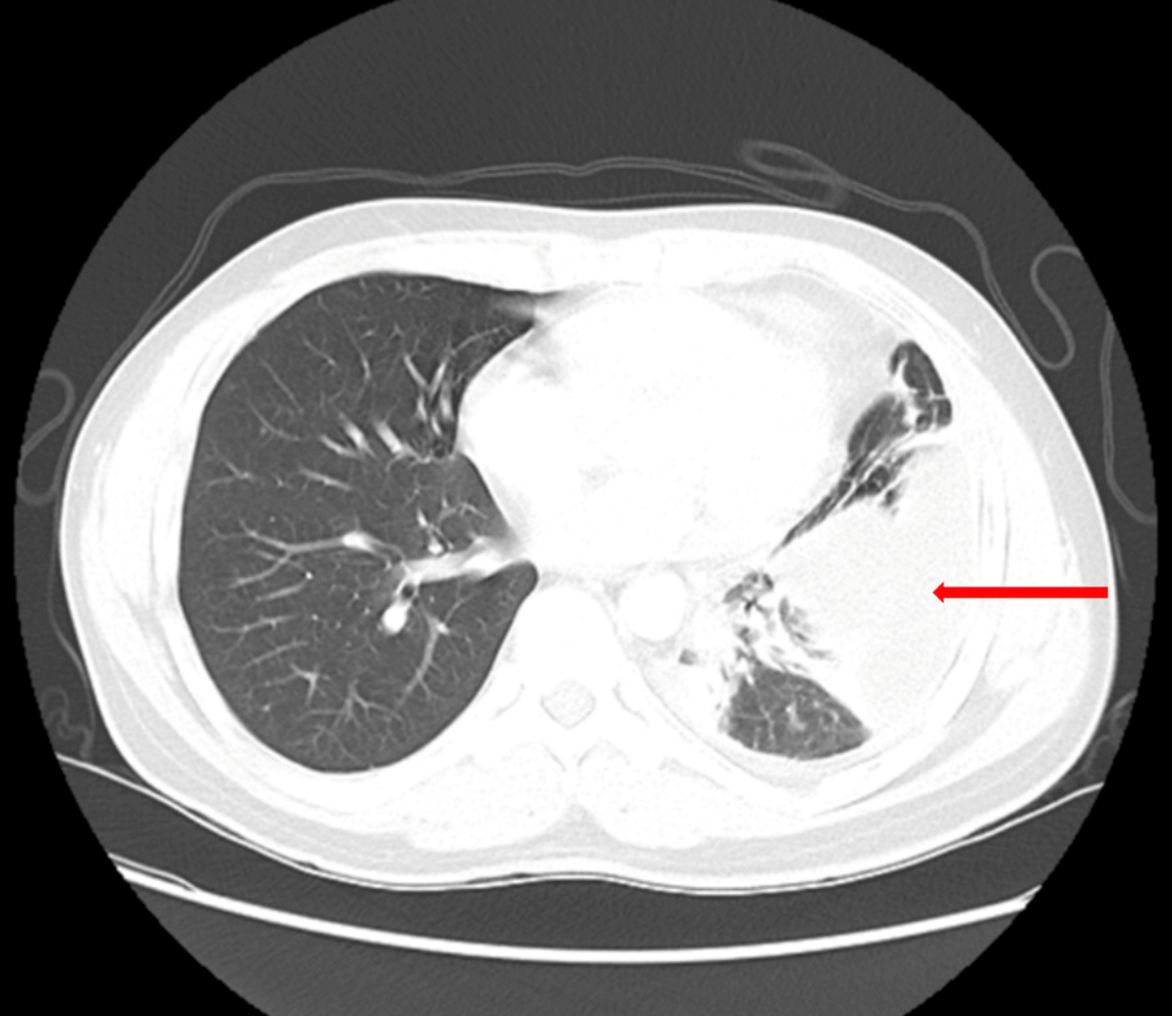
CT of the chest with contrast Red arrow indicating the loculated left-sided pleural effusion/empyema. CT: computed tomography
